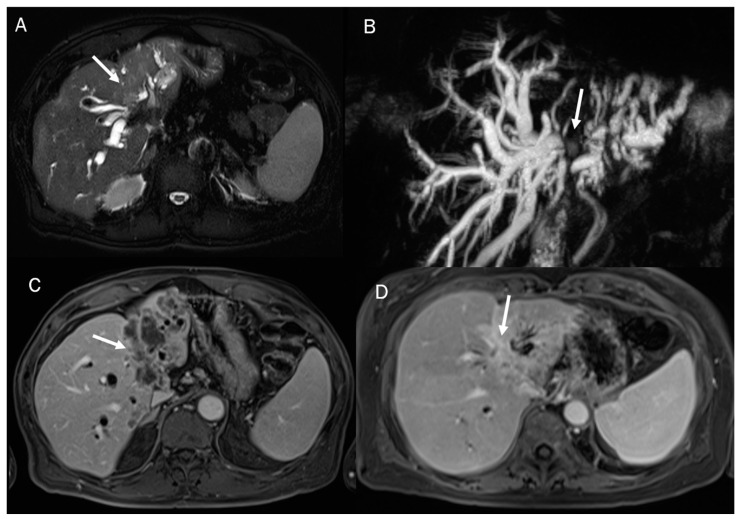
MRI assessment of periductal-infiltrating CCA. The lesion (arrow) shows hyperintense signal in T2-W (A), causing biliary tree dilatation in cholangiography sequences (B). During arterial phase (C), the lesion causes hyperenhancement of surrounding liver parenchymal, showing a progressive contrast enhancement in portal phase (D).
